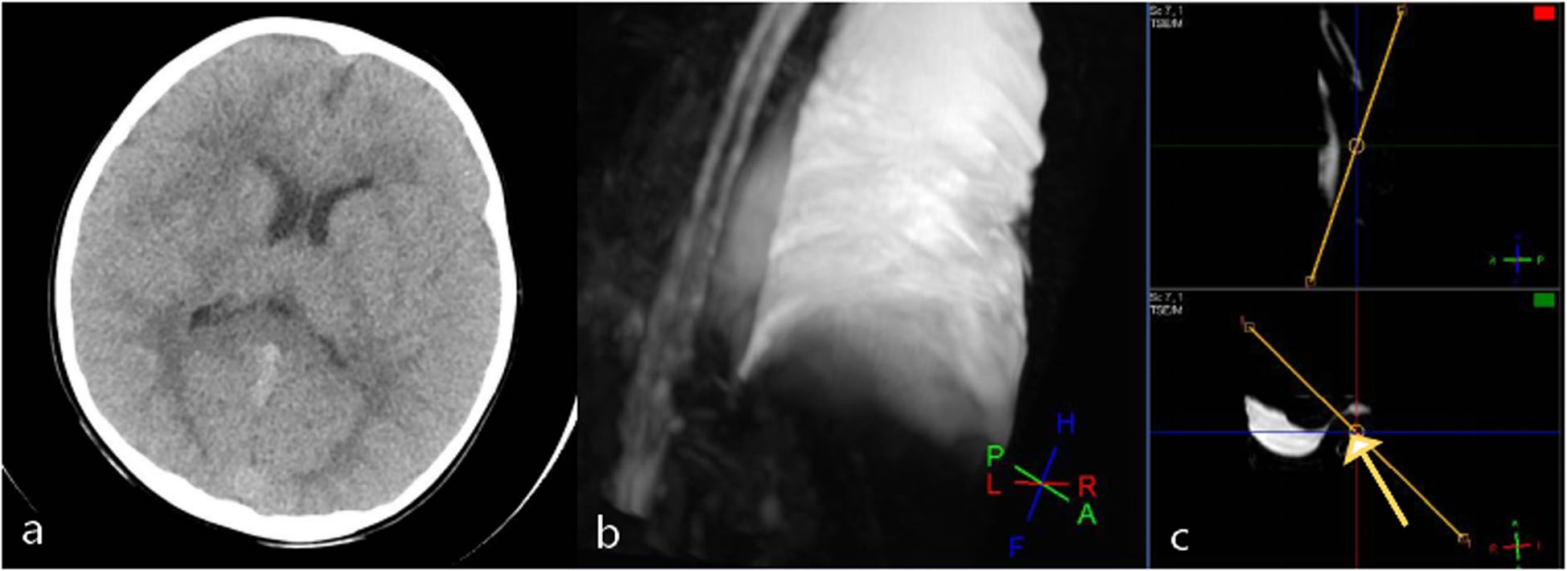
a–c Brain CT and heavily T2 spine MR. a No abnormal signs on brain CT. b, c The arrow in heavily spine MR shows the suspected fistula signal between at T11 level
